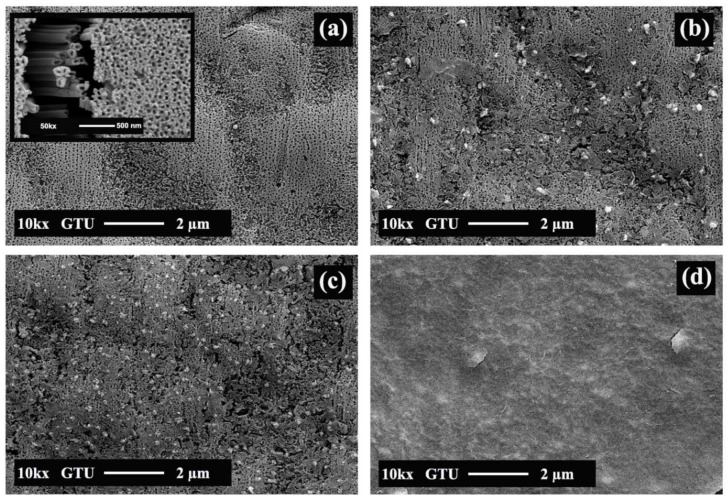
SEM images of (a) pristine TiO_2_ NTs, (b) CT-1, (c) CT-2, and (d) CT-3.
